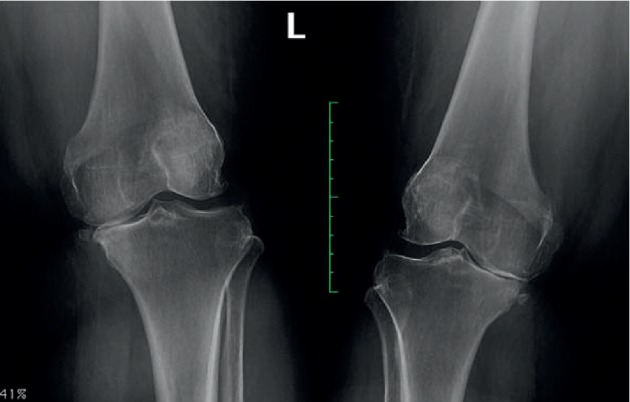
X-ray examination in case 4.
